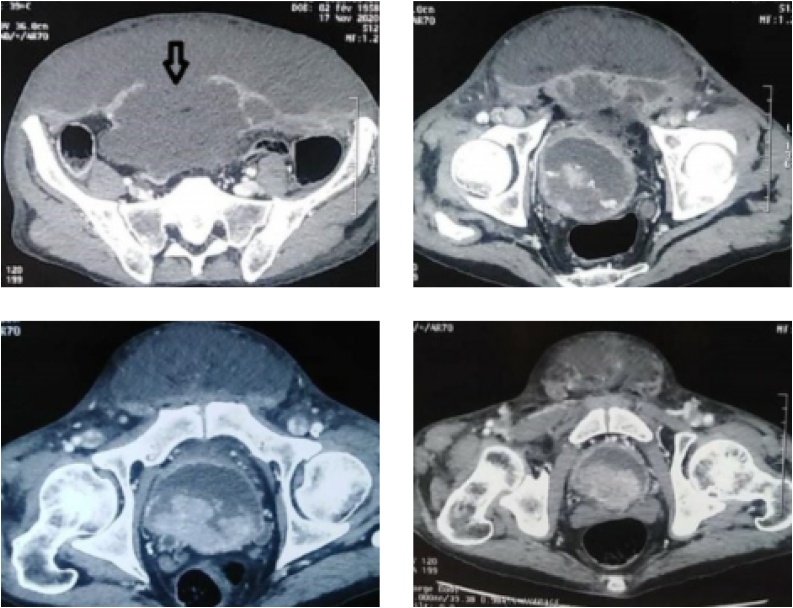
Initial CT scan showing bladder rupture on its anterior wall, large extraperitoneal urinoma and bladder tumour located on its posterior wall.
